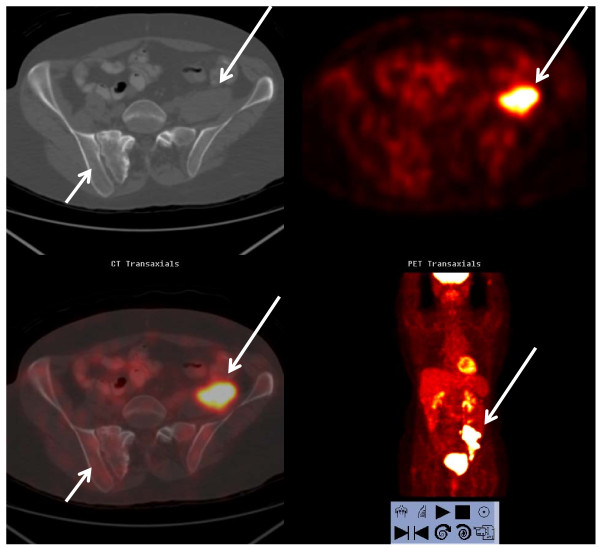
FDG PET/CT scan of the same patient in Figure 1 revealed no focally increased FDG uptake or suspicious abnormalities to bone on the CT of the right sacroiliac area to correspond the bone scan findings (short arrows). The patient's history of pelvic fracture probably explained the focal tracer uptake in bone scan. The recurrent colon cancer in the left pelvis observed by both PET and CT scans is marked by the long arrows.
